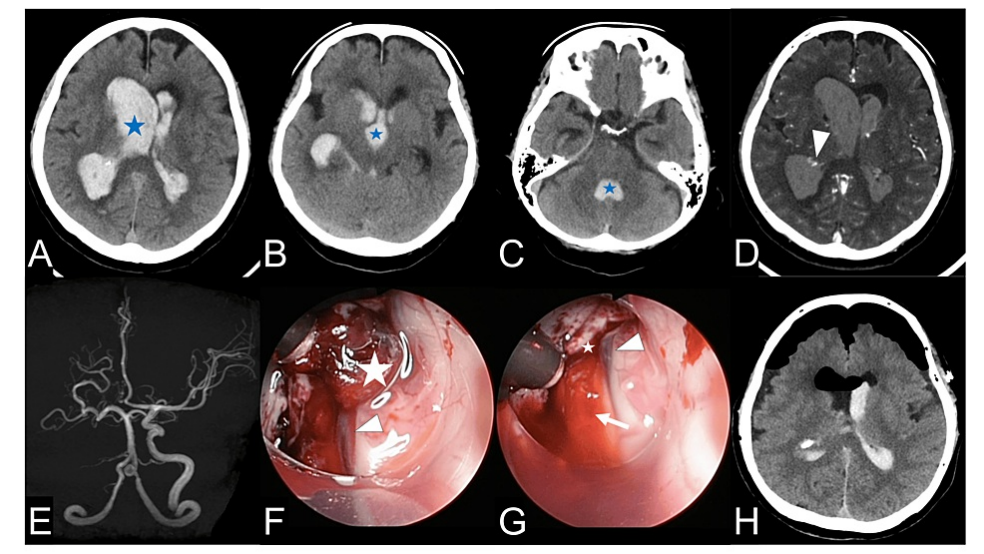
Pre, intra, and postoperative images. A-C: The initial axial non-contrast computed tomography (CT) shows a massive intraventricular hematoma (star) mainly located in the right lateral ventricle (A) extending to the third (B) and fourth ventricle (C). D: Contrast CT shows spot signs (arrowhead) in the posterior horn of the right lateral ventricle, indicating the source of hemorrhage. E: Magnetic resonance angiography shows the occlusion of the right internal carotid artery. F: Intraoperative images in the right lateral ventricles. After removing the hematoma in the right lateral ventricle, the hematoma in the third ventricle (star) was suctioned via the foramen of Monro. The arrowhead indicates the thalamostriate vein. G: Final operative view. The arrowhead indicates the thalamostriate vein, whereas the arrow points to the choroid plexus, and the star indicates the foramen of Monro. H: Postoperative CT shows the sufficient removal of hematoma.
